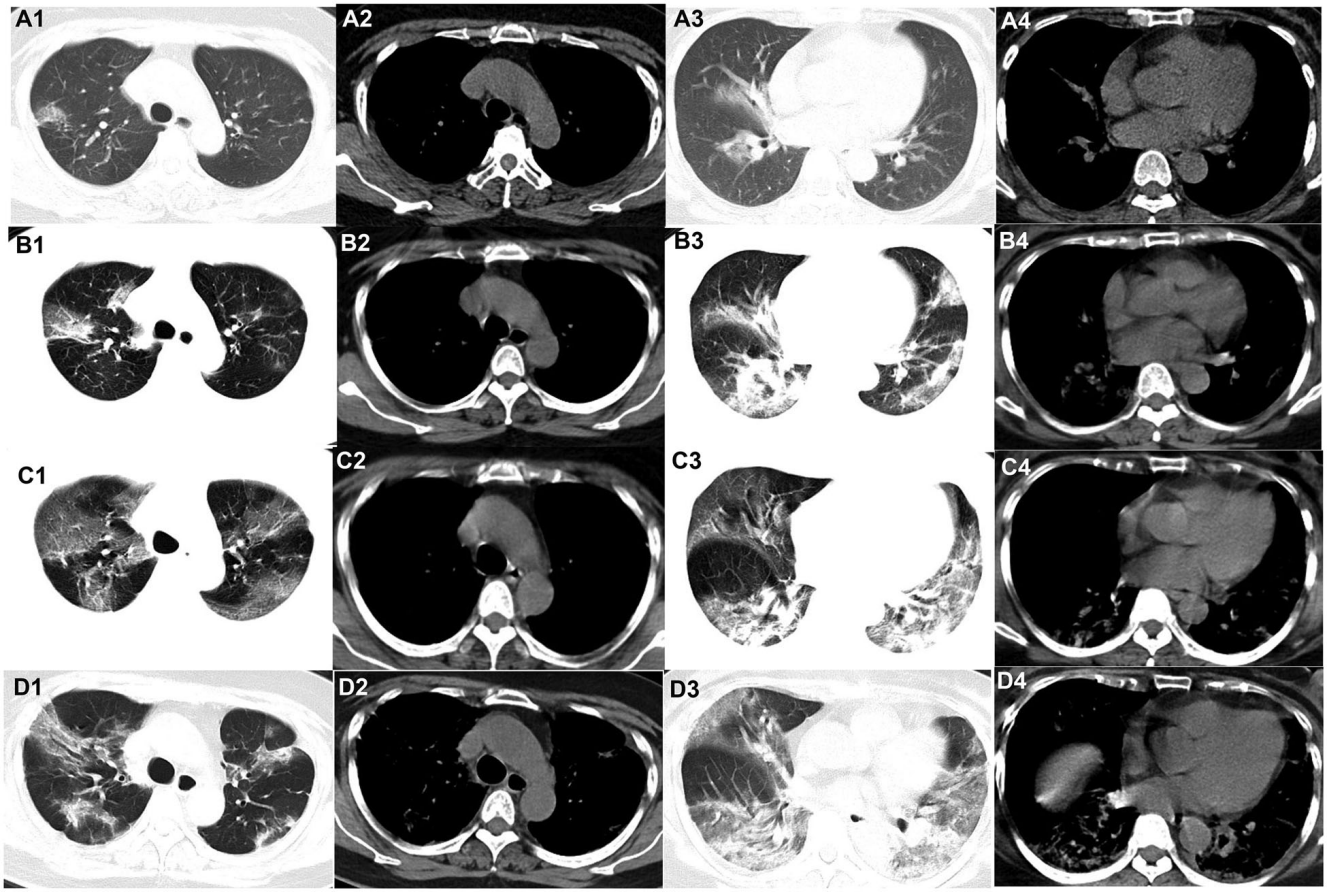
Evolution of the pure ground-glass opacities (GGO) on chest CT of patients with COVID-19. A 58-year-old woman who had close contact with individuals in Wuhan presented with fever for 2 days. (A1–A4) The first non-contrast-enhanced chest CT reveals multipleground-glass opacities in the right upper, middle, and lower lobes (initial chest CT). (B1–B4) Follow-up chest CT 4 days after the first shows that both the scope and density of the lesions increase (stage I*). (C1–C4) Follow-up chest CT 8 days after the first shows that the scope of the lesions increases while the density decreases (stage II*). (D1–D4) Follow-up chest CT 13 days after the first shows that the scope of the lesions decreases slightly while the density increases (stage III*). *The stage does not represent the course of COVID-19 but the time interval between the two adjacent CT scans.
